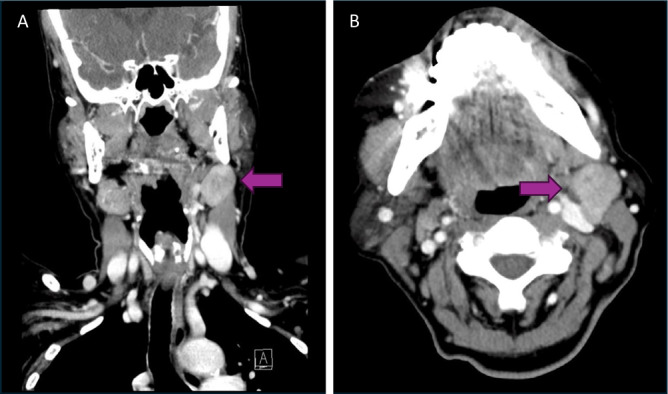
CT neck with contrast in coronal (A) and transverse (B) demonstrating a 2.2 x 2.3 x 3.2 cm heterogeneous mass between left submandibular gland and left SCM.
